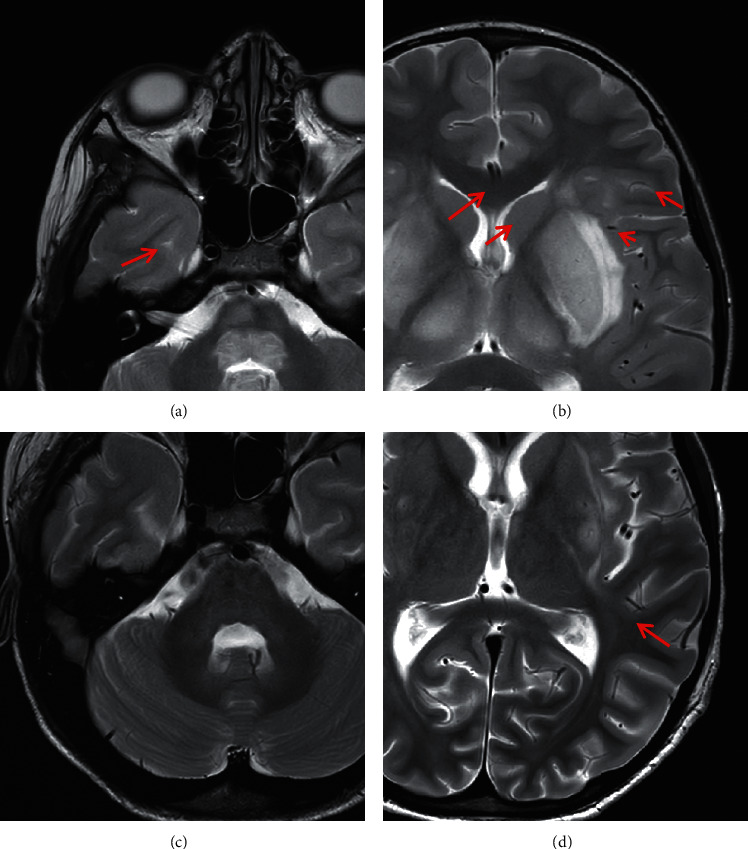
MRI scan at presentation (a, b) and at two weeks follow-up (c, d). In a and b, axial T2 weighted images show hyperintense signal changes in the tegmentum of the pons (a, red arrow), in the thalami (b, red arrows), in the putamen bilaterally with involvement of external capsule, claustrum, and capsula extrema (b, red arrows), more prominent in the left side. In c and d, the follow-up MRI scan shows a regression of the signal changes with the exception of the persistence of a small area of hyperintensity in the left putamen and external capsula (d, red arrow).
